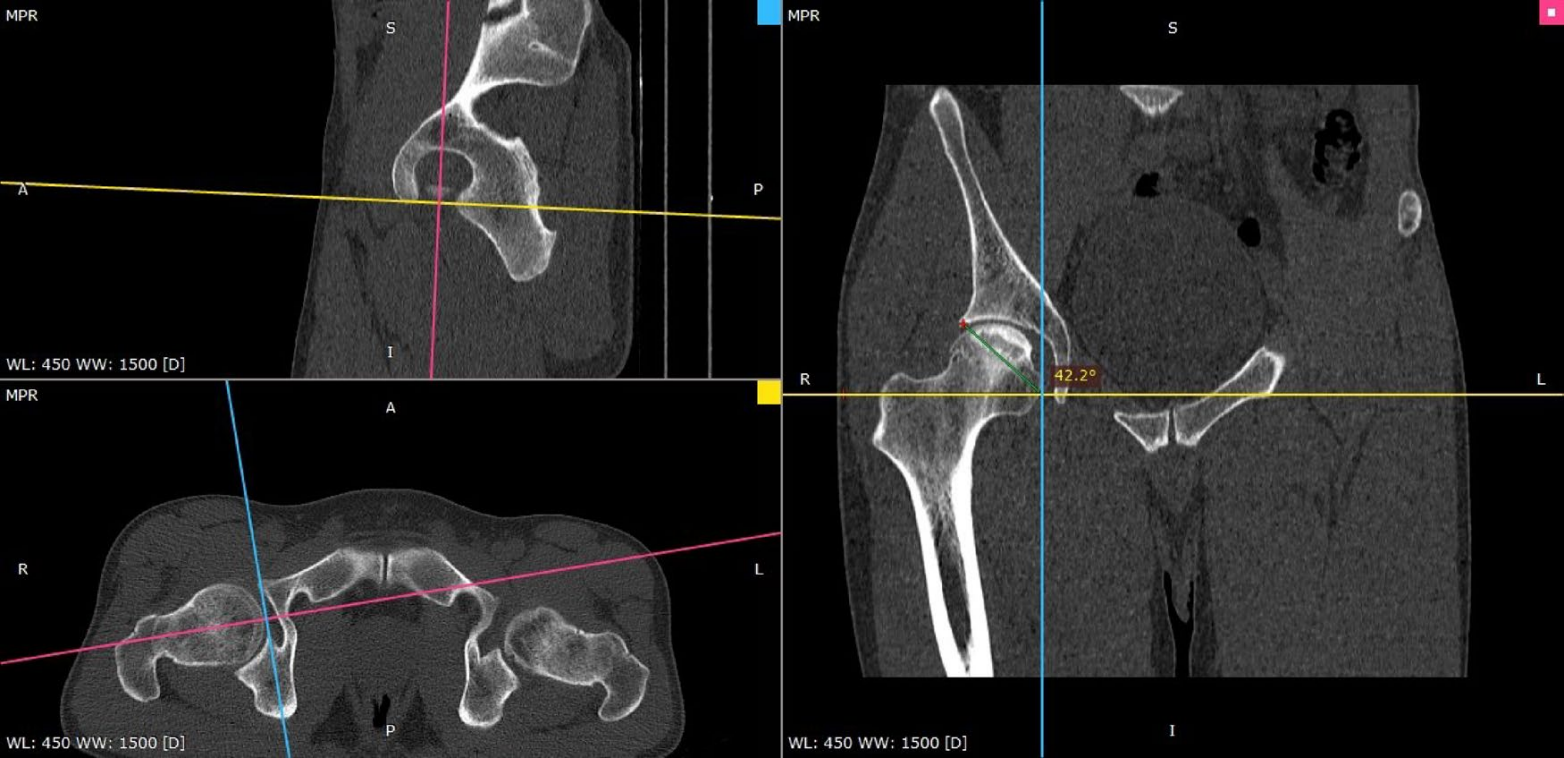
Preoperative determination of TAL and measurement of TAL inclination angle by CT-MPR^4^.
